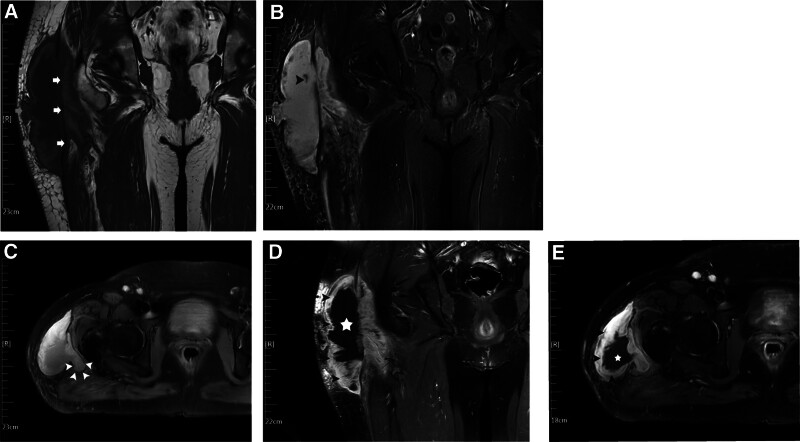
(A) T1-weighted coronal image presents an irregular soft tissue mass with mixed signals between the adipose layer and the deep fascia of the right hip, demonstrating an isohigh signal, partially engaging the deep tissue, and spanning approximately 185 mm in length; gray arrows: adipose layer; white arrowheads: deep fascia. (B) STIR sequence reveals adipose tissue signal suppression, while certain regions within the lesion exhibit a low signal (short gray arrow), indicative of fat particles. (C) STIR in the transverse section elucidates that the lesion penetrates the deep fascia and extends into the deeper tissues; short white arrows: the lesion penetrating the deep fascia. (D, E) Coronal and cross-sectional enhancement images display perilesional enhancement with a low signal centrally and encroachment of the lesion into both the fat layer and the deeper strata of the deep fascia; short black arrows: enhancement of the perilesional tissue; white pentagrams: low-signal central region of the lesion. STIR = short tau inversion recovery.
